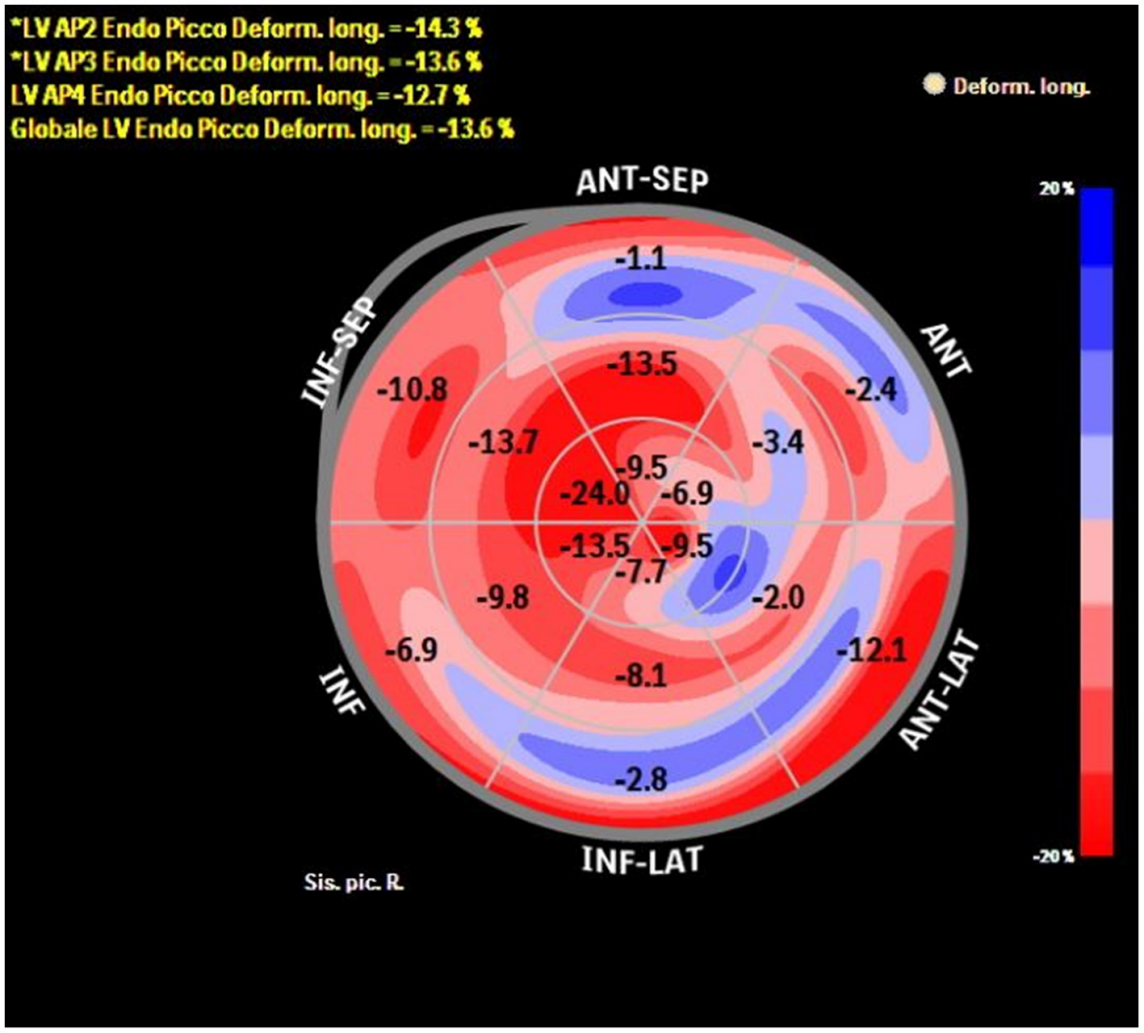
Bull-eye depicting longitudinal strain of the LV by speckle tracking echocardiography in a patient with previous TTS at 1-month follow-up after the acute event. Despite complete recovery of LVEF, longitudinal systolic function is still impaired (global longitudinal strain −13.6%).
